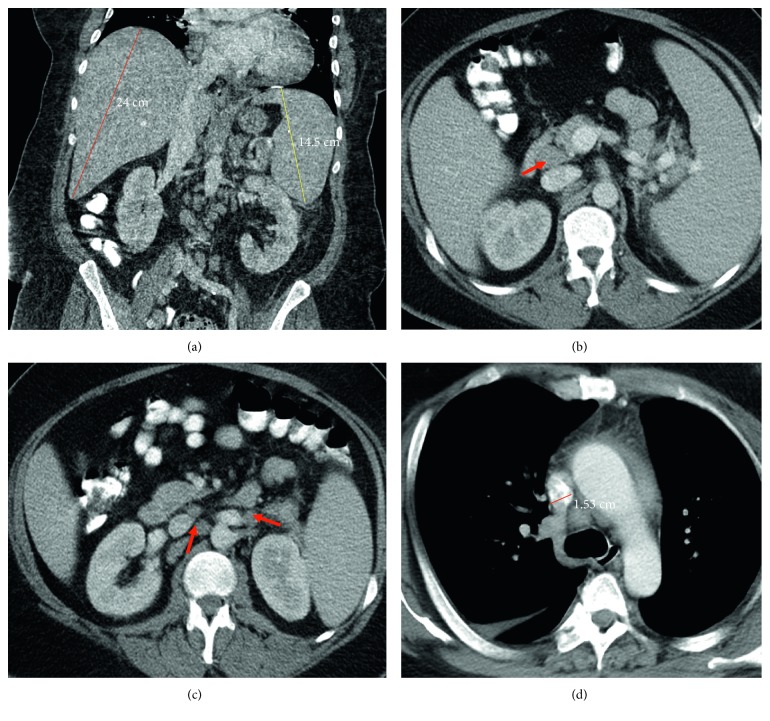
The abdomen and thoracic CT scan. (a) Coronal contrast-enhanced CT image of the abdomen with hepatosplenomegaly: the craniocaudal diameter of the right liver lobe is 24 cm (normal 12–17 cm); the craniocaudal diameter of the spleen is 14.5 cm (normal up to 13 cm). (b) Enlarged mesenteric lymph nodes (red arrow) measure 1.6 cm in short axis. (c) Para-aortic lymph nodes (red arrows) measure about 1.4 and 1.3 cm in short axis. (d) Lymph node in mediastinal station 4R measures about 1.5 cm in short axis.
